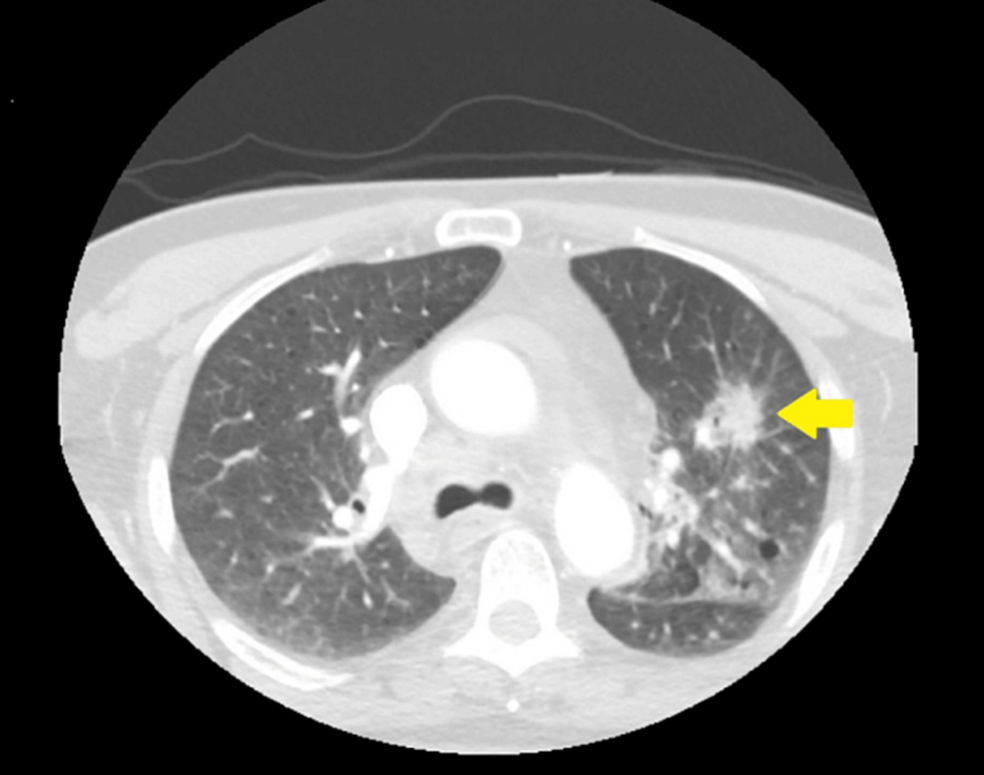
CTA of the neck showing a spiculated mass lesion affecting the left upper lobe, with adjacent patchy airspace opacities in the left mid-lung field, along with prominent mediastinal adenopathy CTA, CT angiography
